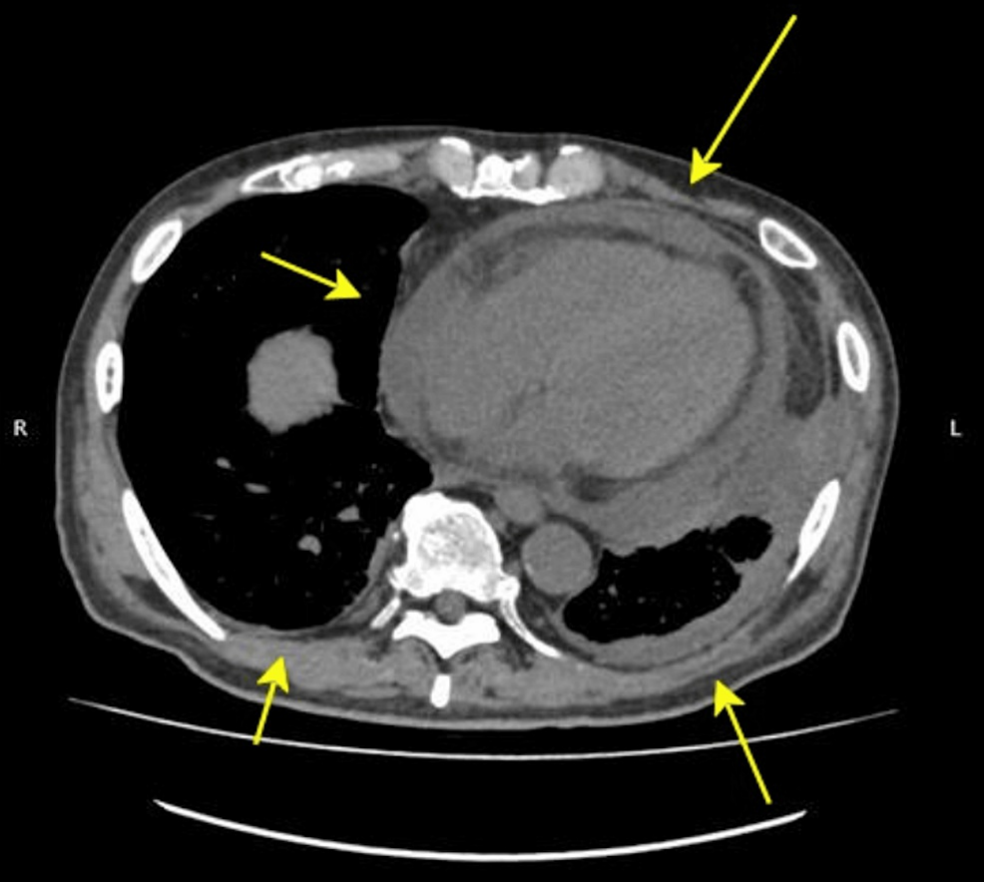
Chest computed tomography revealed bilateral pleural effusion and pericardial effusion.
